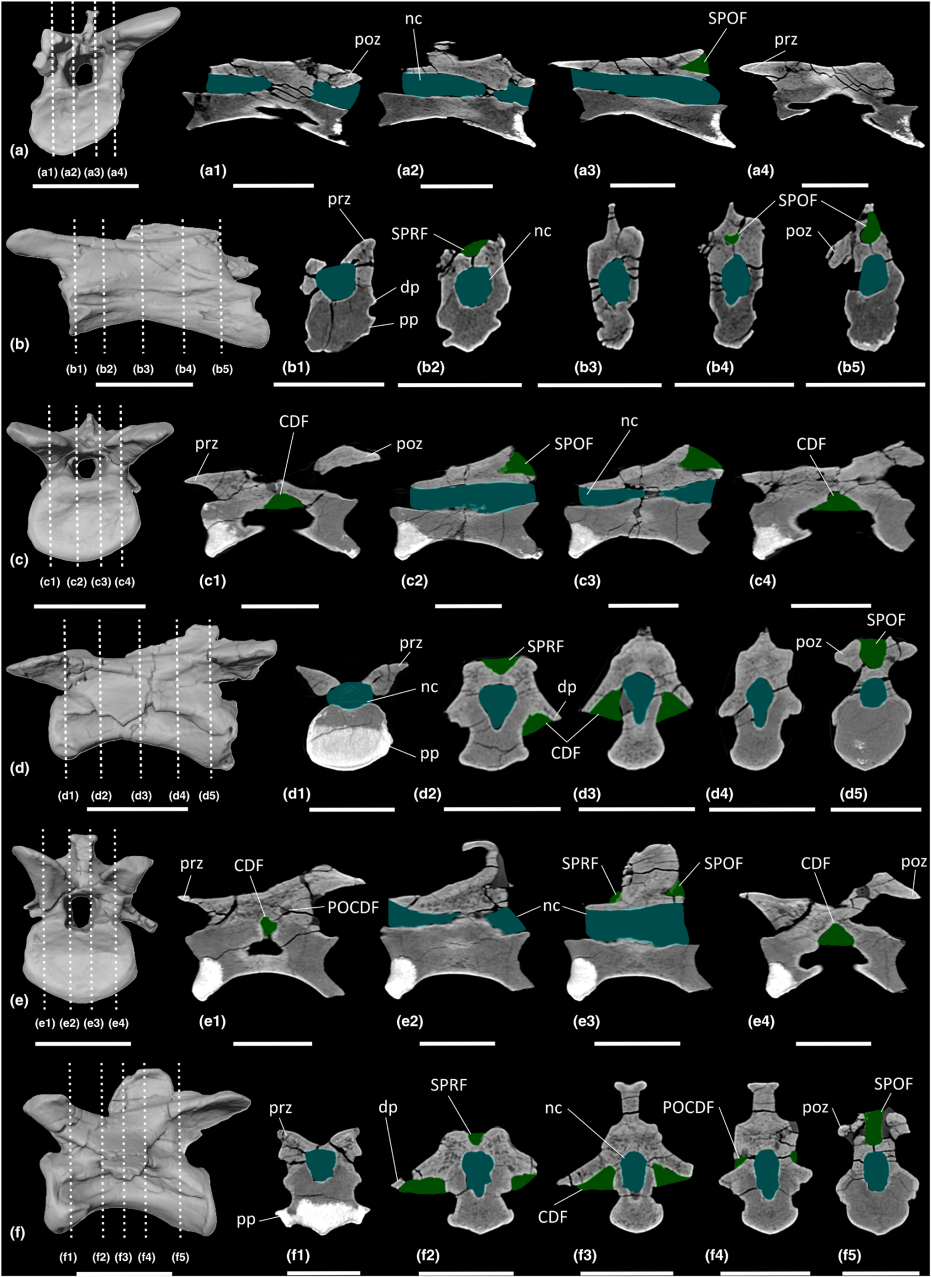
CT scan data of *Plateosaurus longiceps* MB.R.4404 ‘Skeleton 25’ cervical vertebrae. (a, b) cervical vertebra 4 MB.R.4404.18, (c, d) cervical vertebra 7 MB.R.4404.21, (e, f) cervical vertebra 9 MB.R.4404.23; (a, c, e) surface scan anterior view, (b, d, f) surface scan lateral view; (a1–a4, c1–c4, e1–e4) CT scan slices in parasagittal cross‐section, (b1–b5, d1–d5, f1–f5) CT scan slices in transverse cross‐section. CDF, centrodiapophyseal fossa; dp, diapophysis; nc, neural canal; POCDF, postzygapophyseal centrodiapophyseal fossa; poz, postzygapophysis; pp., parapophysis; prz, prezygapophysis; SPOF, spinopostzygapophyseal fossa; SPRF, spinoprezygapophyseal fossa. Key: Blue highlight, neural canal; green highlight, fossa. Scale bars = 5 cm.
